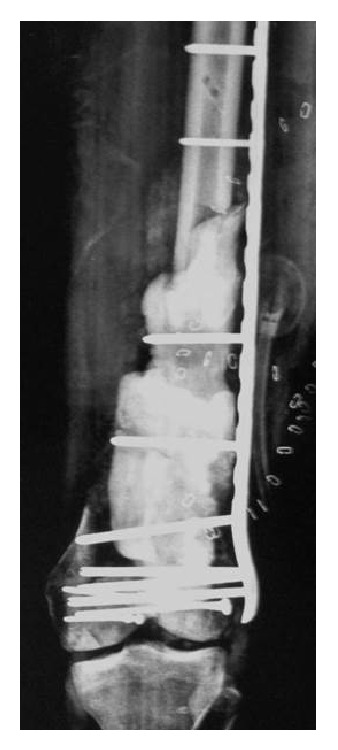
Radiograph following placement of antibiotic cement spacer with lateral distal femoral locking plate fixation.
